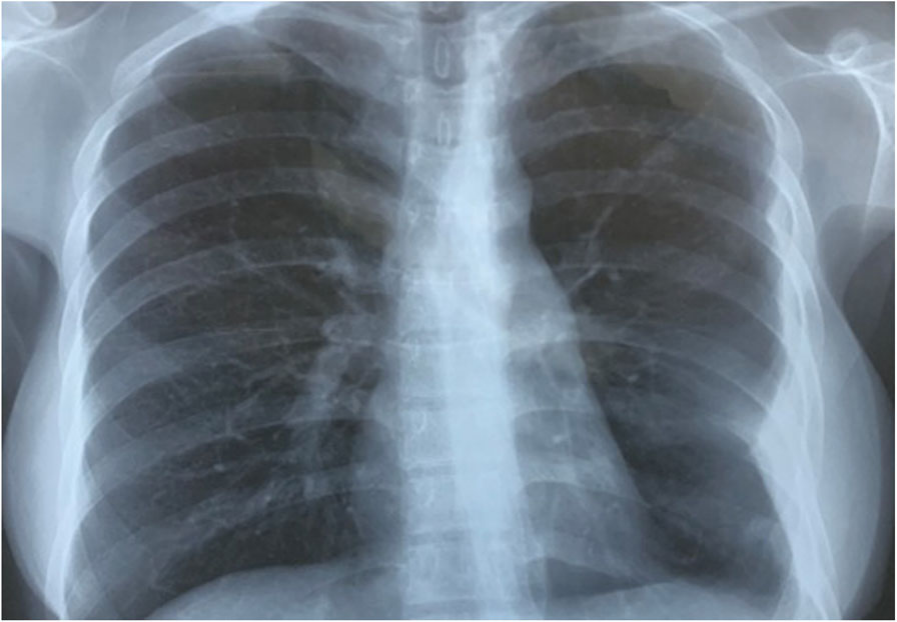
Posteroanterior chest radiograph showing miliary shadows in both lung fields
